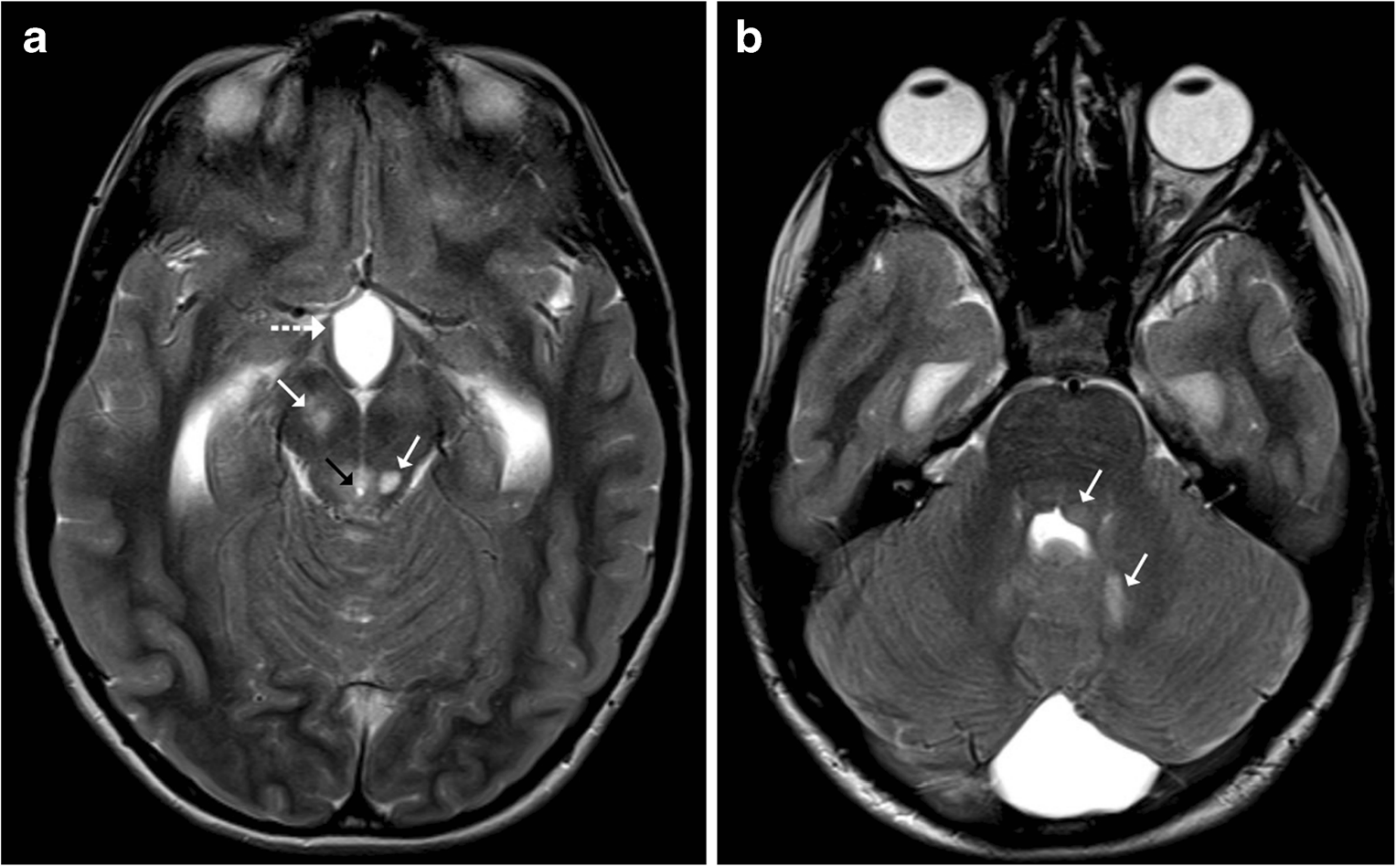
A known case of neurofibromatosis type I with hydrocephalus: a–b Axial T2-weighted images show mild expansion of the midbrain and pons with multiple hyperintense lesions involving the midbrain, pons and cerebellar peduncle (*arrow*). Periaqueductal hyperintensity with narrowing of the aqueduct (*black arrow*) resulting in third ventricular dilatation (*dotted arrow*)
